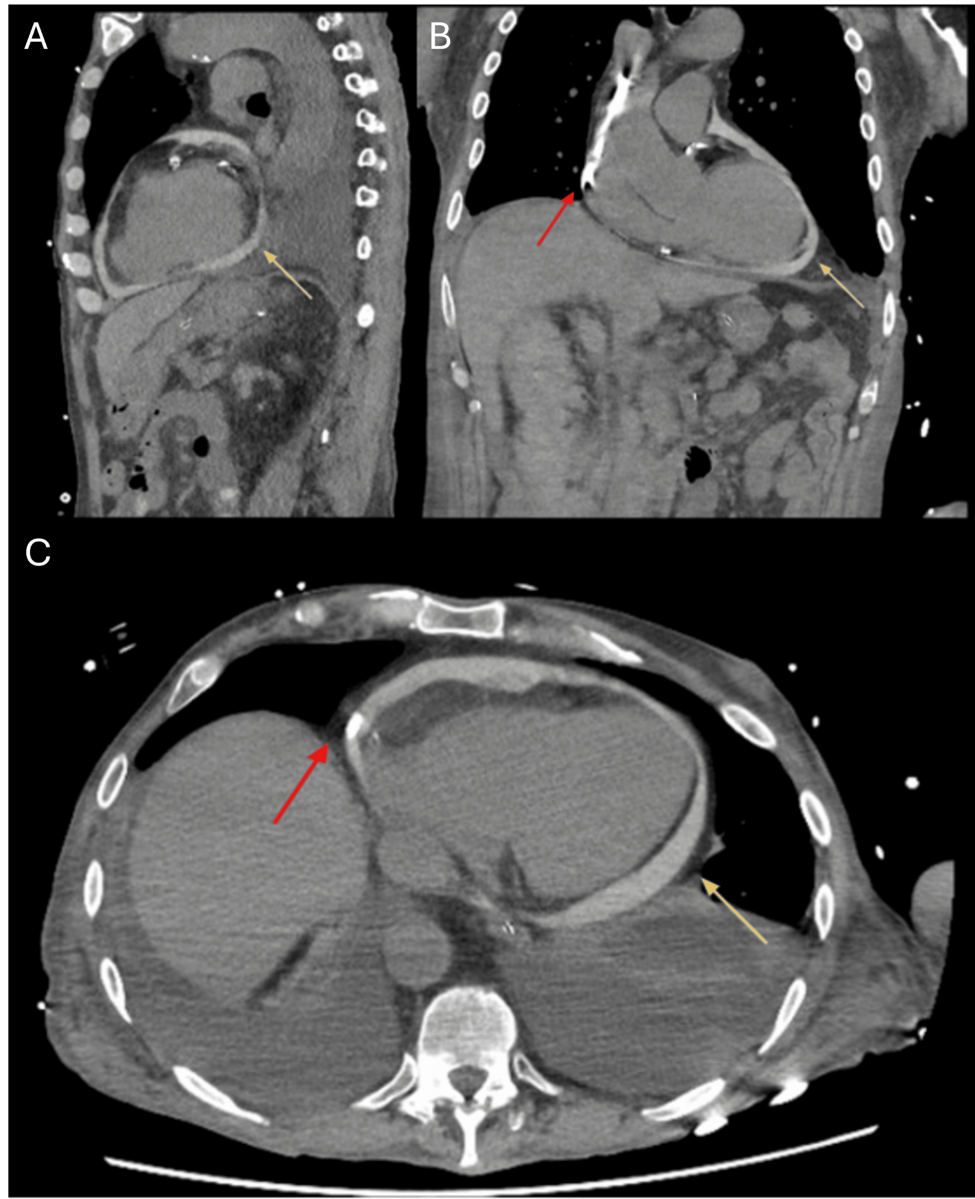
Contrast-enhanced CT via the catheter. (A-C) Contrast-enhanced CT via the catheter (red arrow) showing an increase in pericardial effusion (yellow arrow) compared to the previous scan.
